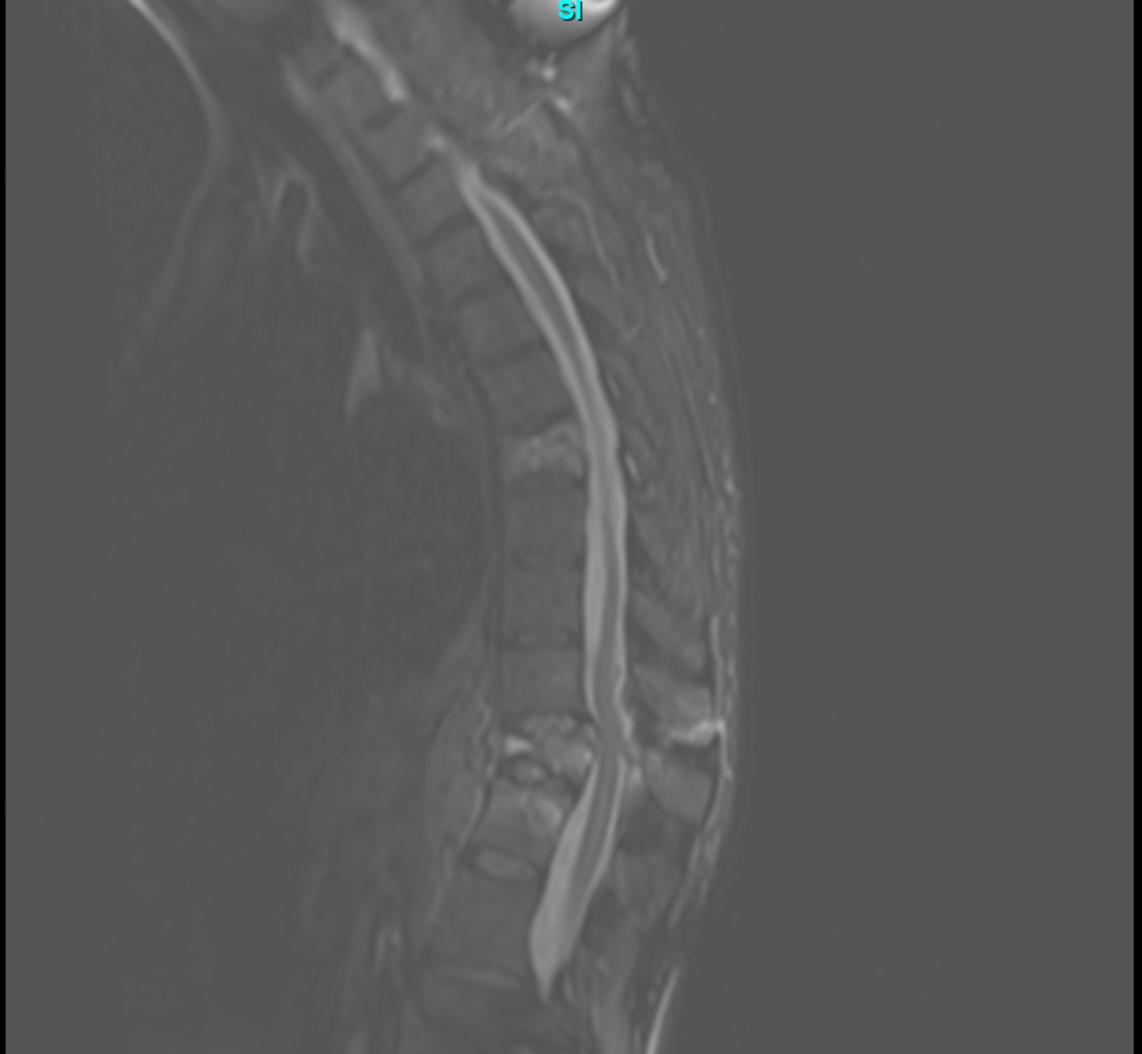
Pre-operative thoracic magnetic resonance imaging (MRI) demonstrating T11 burst fracture
